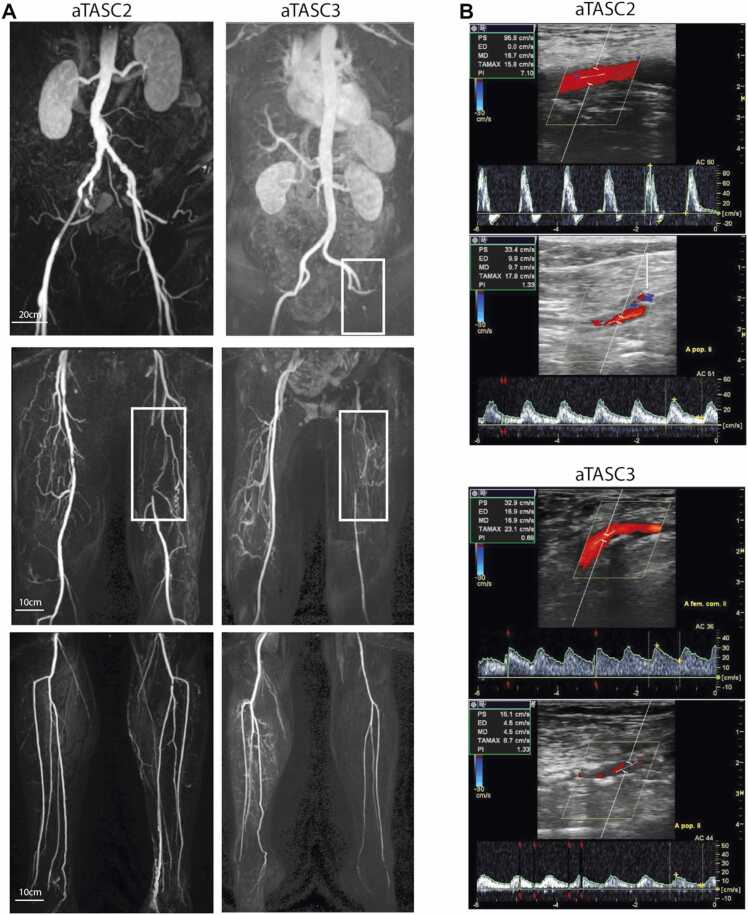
Representative imaging results. A Representative magnetic resonance angiography (MRA) images from an aTASC 2 (left) and aTASC 3 (right) patient. Top images: aorto-iliac (AI) vessel section, middle images: femoro-popliteal (FP) vessel sections and lower images: infrapopliteal (IP) vessel sections. White boxes outline detected stenoses. B Representative color-coded duplex sonography (CCDS) images from an aTASC 2 (top images) and aTASC 3 (bottom images) patient. The top image shows the flow profile and the measured peak systolic velocity (PSV) of the common femoral artery (CFA), respectively, and the bottom image of the popliteal artery (PA). The aTASC 2 images show a biphasic flow profile in the CFA and a monophasic flow profile in the PA, and the aTASC 3 images show a monophasic flow profile in the CFA and PA.
